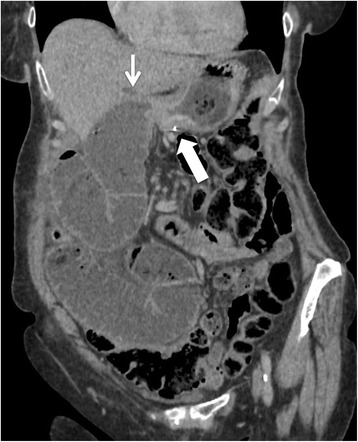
CT without contrast injection: frontal reconstruction showing gastric wall thickening at the gastrojejunostomy site (large arrow). The afferent jejunal loop is dilated with upstream biliary dilatation (thin arrow)
